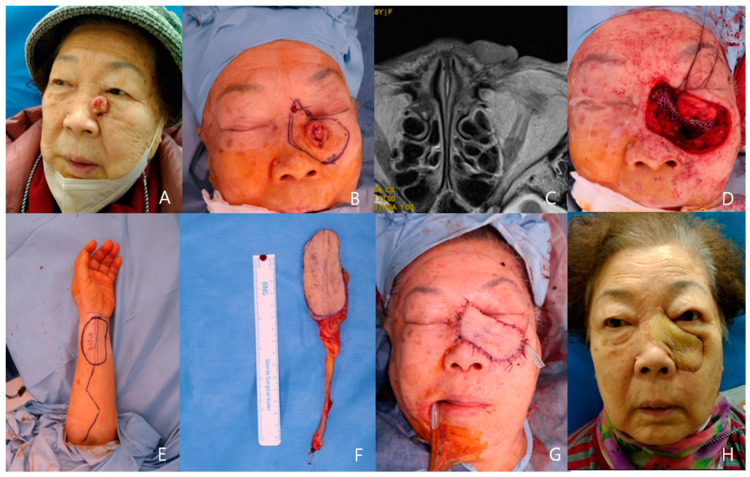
A case of left nasal wall and lower lid squamous cell carcinoma patient. (A) Pre-operative photo. (B) 2cm surgical safety margin. (C) MRI finding: 2 × 2 cm size and 8 mm depth SCC adjacent to bony wall. (D) After En-bloc excision of cancer and bony frame reconstruction using Synpor. (E) Radial forearm flap design. (F) Harvested flap. (G) Immediate postoperative view. (H) Post-operative 3 month view.
